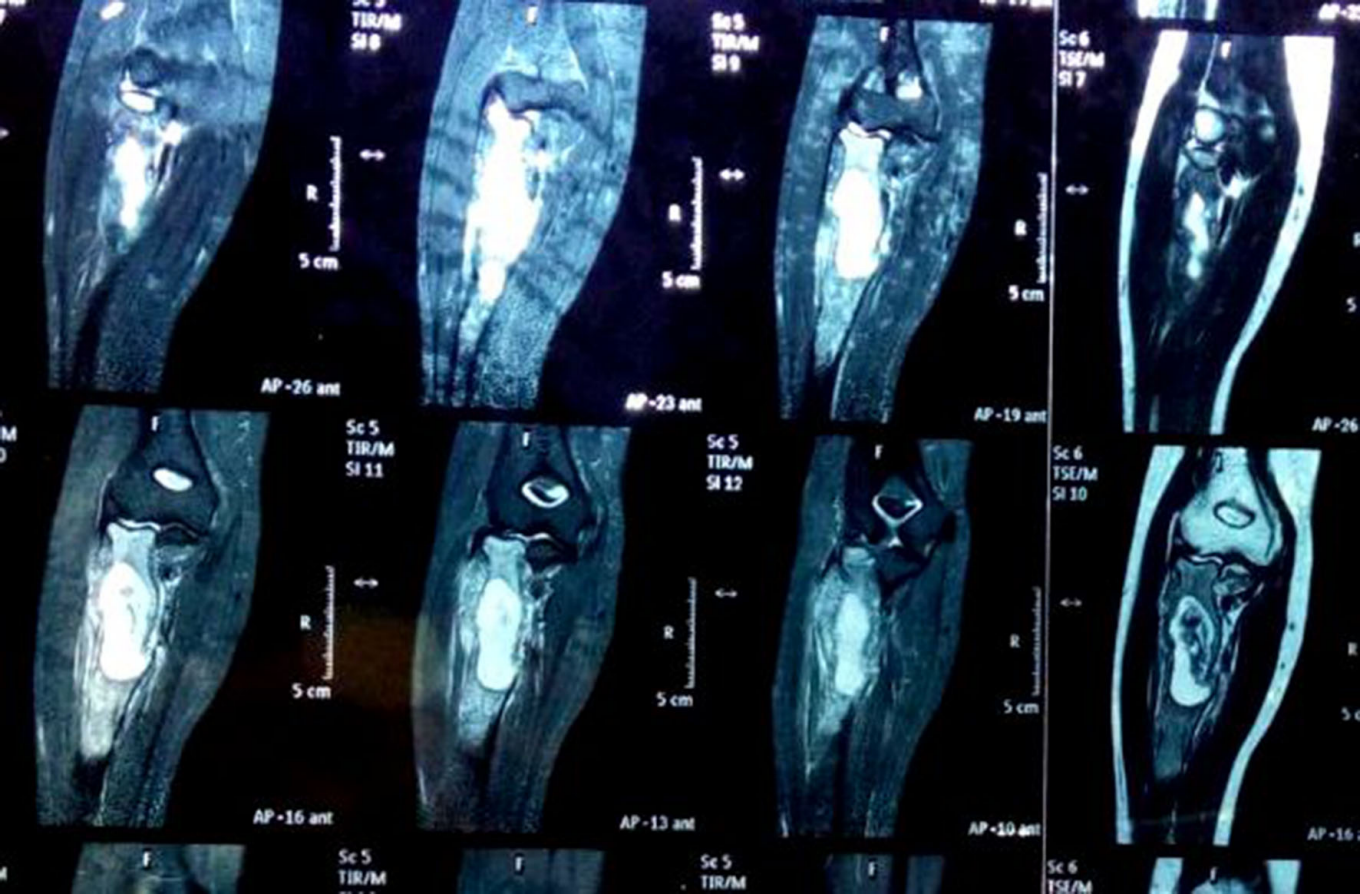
MRI sagittal view with hyperintense areas with lytic areas extending extraosseous
